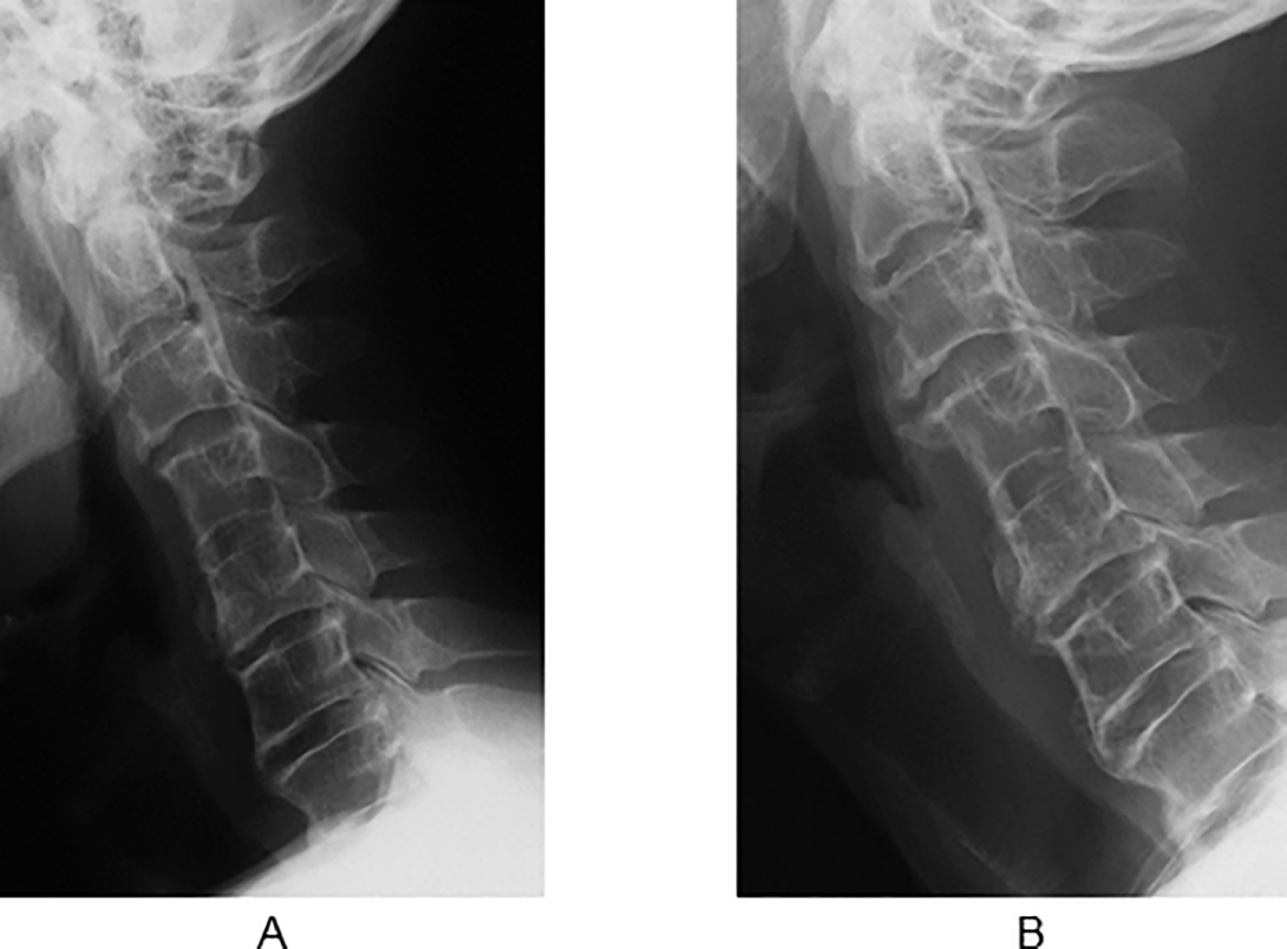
Lateral radiographs of patient no. 4 obtained 9 and 17 years after surgery (long-term follow-up). (A) Nine years after surgery, recurrent anterior osteophytes were observed at the C3/4 and C5/6 levels. (B) Seventeen years after surgery, the osteophytes were gradually enlarged at the C3/4 and C5/6 levels.
